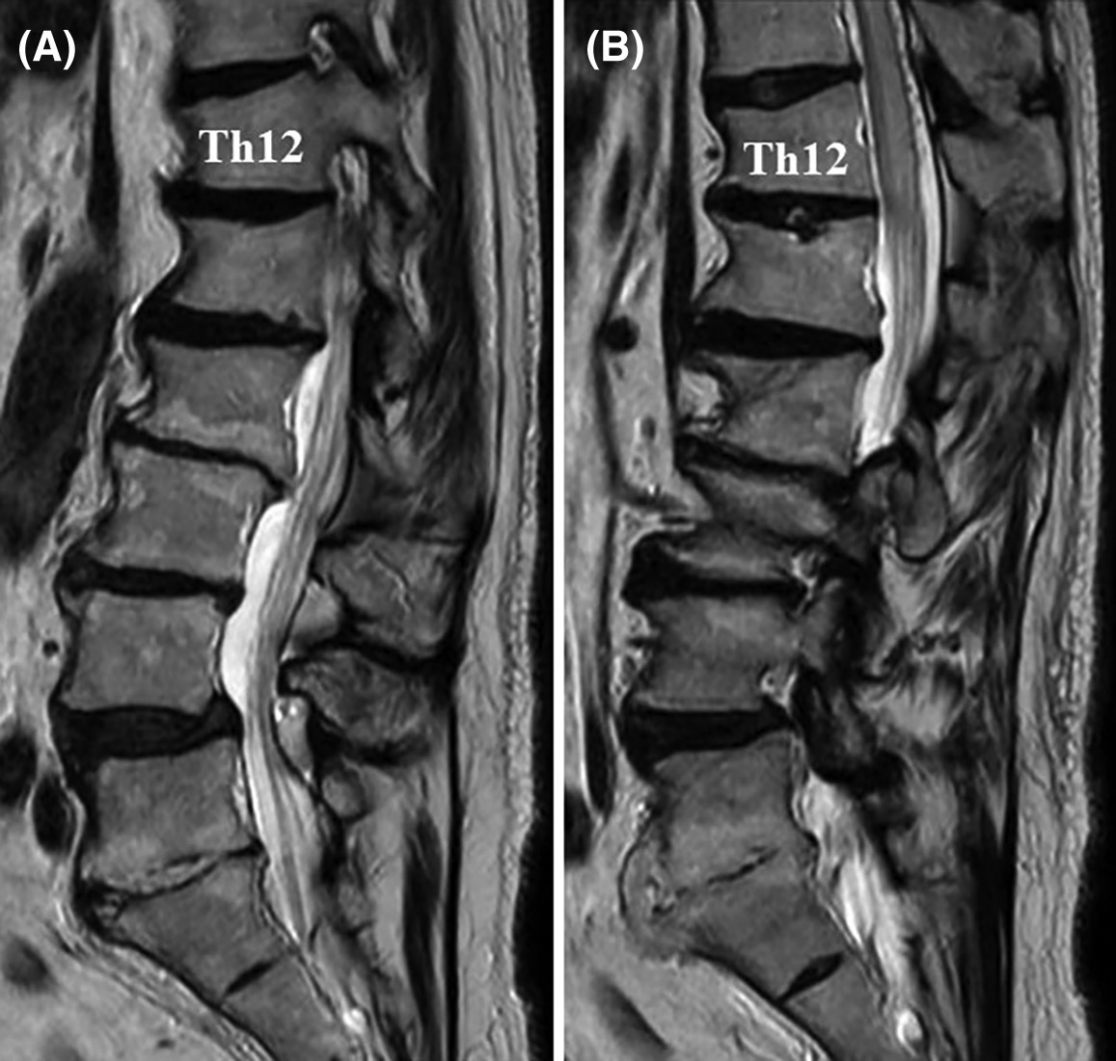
Initial magnetic resonance imaging (MRI) of the lumbar spine.(A, B) Sagittal T2‐weighted MRI showing lumbar spondylosis, spinal cord edema, and flow voids around the cord at the Th11–L1 level.
